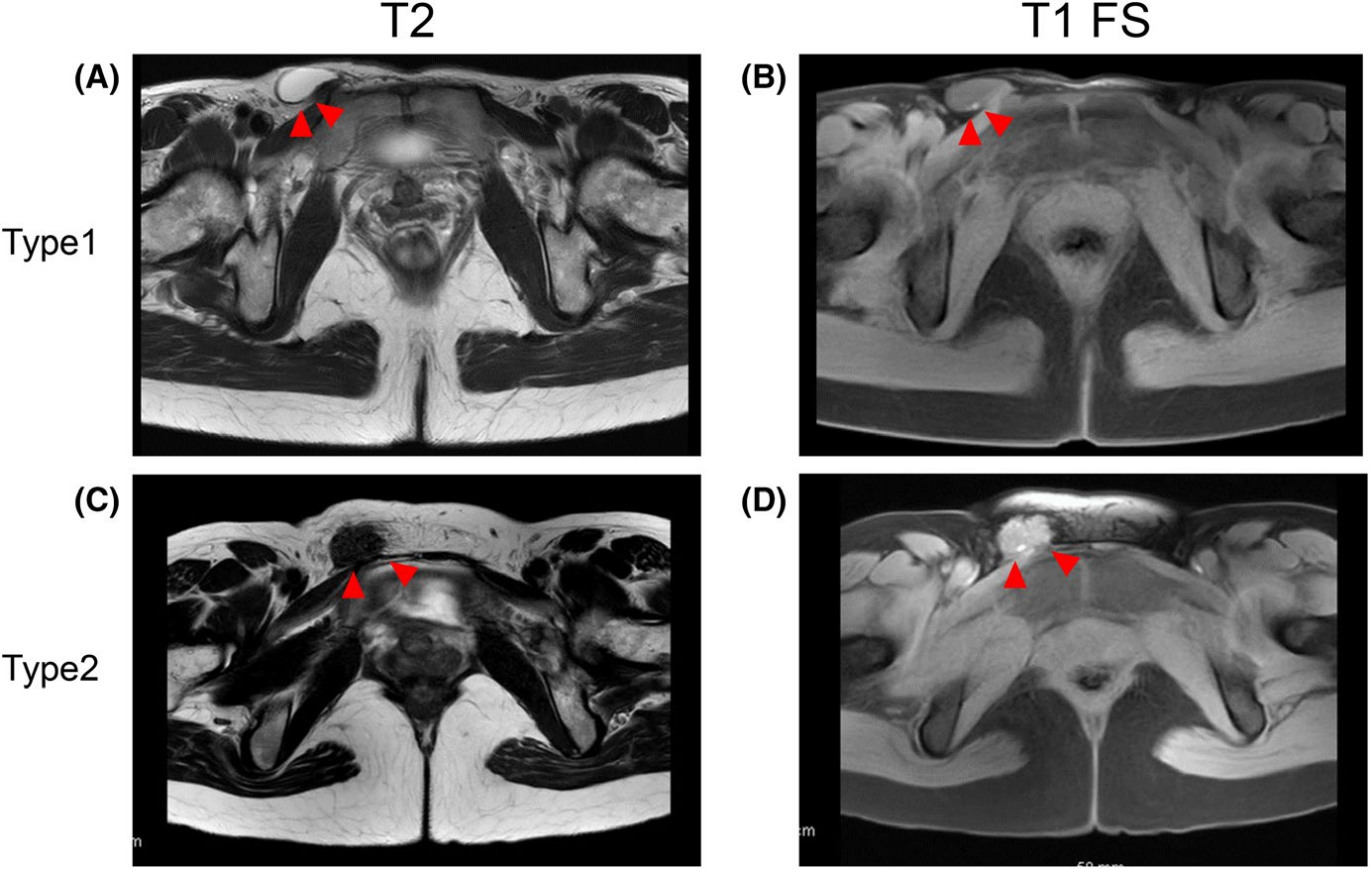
Two types of inguinal endometriosis revealed by magnetic resonance imaging. Red arrowheads denote inguinal endometriosis. A, T2‐weighted axial image shows cystic lesions in the right groin. B, Fat‐saturated T1‐weighted axial image shows the hyperintense nodule in the wall of the cystic lesions. In this case, endometriosis exists and endometriotic lesion exists at the wall of a hernia sac or hydrocele of Nuck’s canal. C, T2‐weighted axial image shows the right inguinal mass (isointense with muscle). D, Fat‐saturated T1‐weighted image shows hyperintensity in the nodule. In this case, endometriotic lesions exist in the solid fibrotic mass
